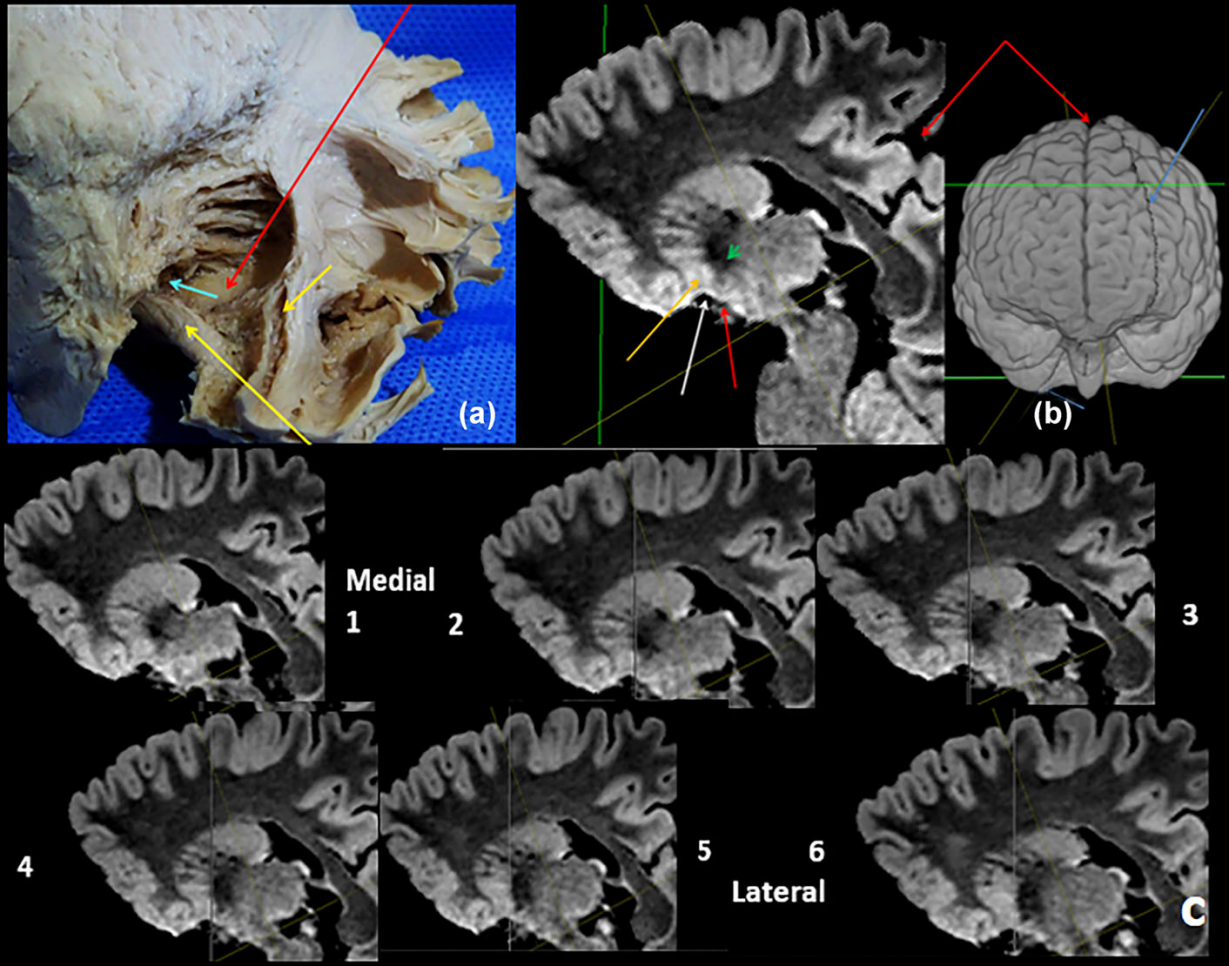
(a) Right hemisphere of Charcot's cut. External capsule and lenticular nucleus resected—light blue arrow, portal for the bridge accumbens putamen; orange arrow, furrow for the claustrum; yellow arrow, anterior commissure; red arrow, floor of the capsule. (b) Oblique MRI cut through the anterior limb in our model with an inclination of 35° in the coronal, and of 25° in the axial sense. The anterior limb is well shown—white arrow, medial anterior perforated substance (APS); orange arrow, accumbens; red arrow, optic tract; short green arrow, anterior commissure; at the side, oblique plane seen upon a 3D reconstruction of the brain of our model (light blue arrows); red arrows, sagittal fissure. See the difference of signal of the frontal and temporal poles with this sequence. (c) MRI in our model. A run of 0.5 mm oblique cuts of the anterior limb of the internal capsule; its breadth imports approximately 3.5 mm. *Note*: The greater intensity of signal of the accumbens.
